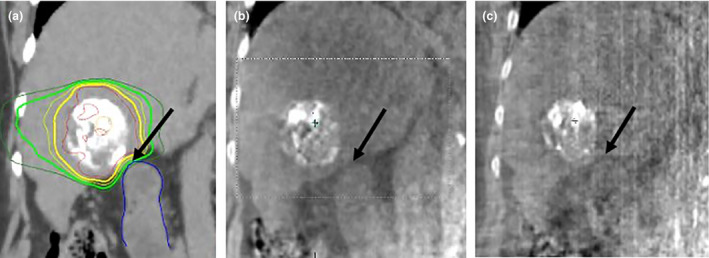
Planning CT (a) showing intra‐tumoural lipiodol with black arrow at region of steep dose gradient at interface between tumour and duodenum (blue contour). Adequate visualisation of tumour–duodenum interface is maintained with pre‐treatment CBCT (b) and intrafraction volumetric imaging (IF‐CBCT) (c) allowing accurate placement of steep dose gradient
